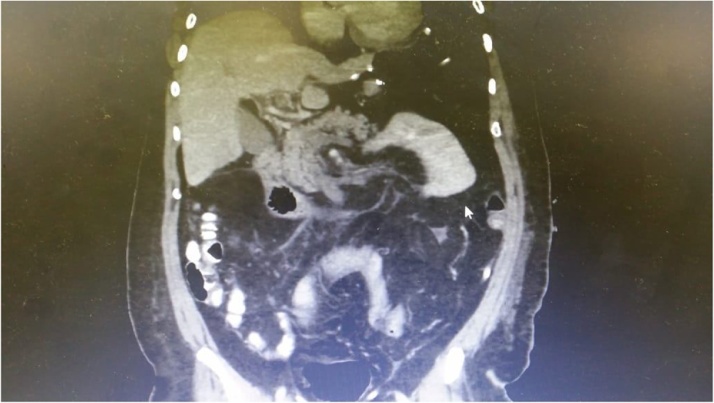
Computed tomography (CT) of the abdomen showing portal vein thrombosis without evidence of small bowel ischemia.
